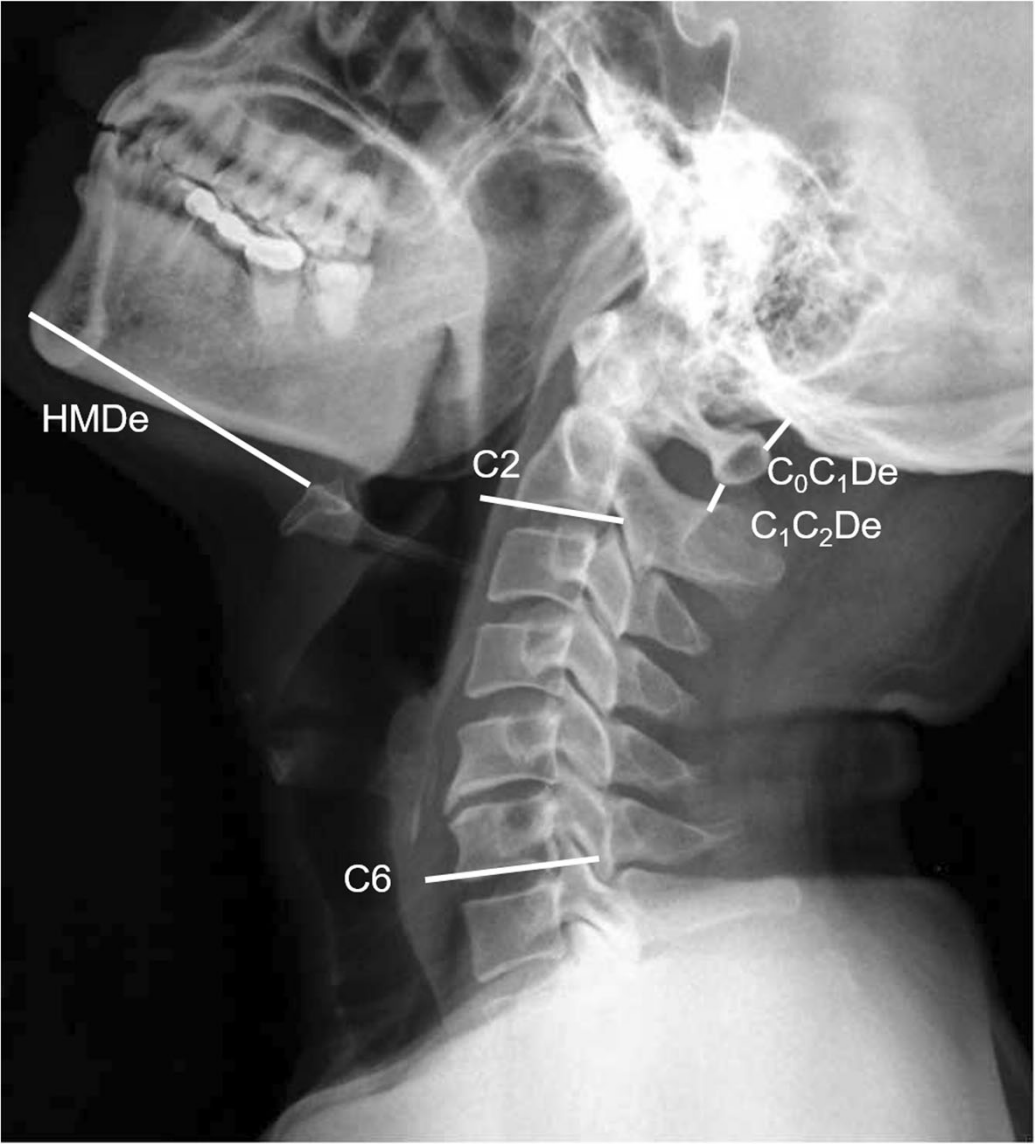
Lateral cervical X-ray film in the extension positions. HMDe, the distance between the hyoid bone and the tip of the chin in the extension position; C_0_C_1_De, the distance between the occipital bone and first cervical vertebra in the extension position; C_1_C_2_De, the distance between the first cervical vertebra and the second cervical vertebra in the extension position
